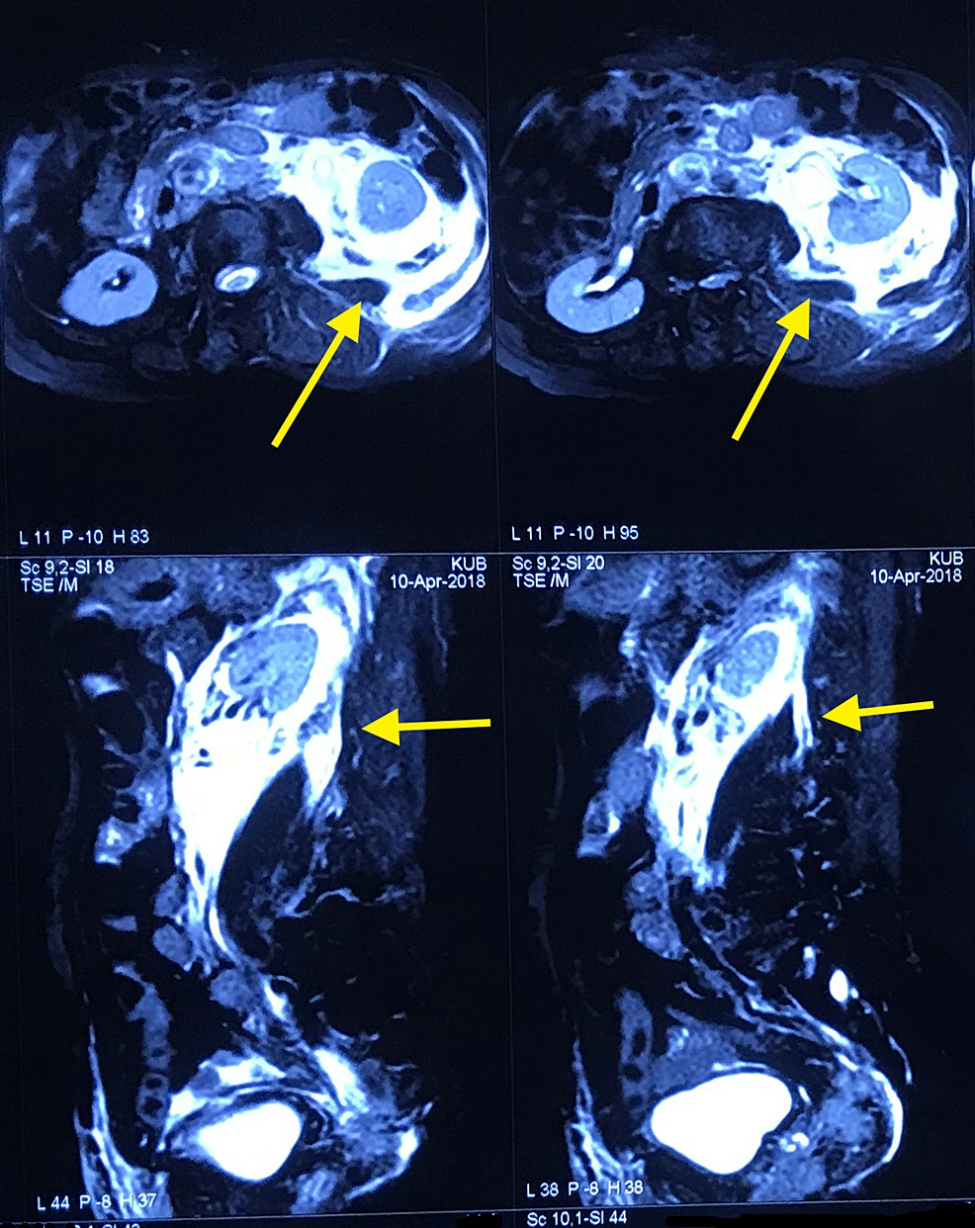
MRI imaging depicting the urinoma
